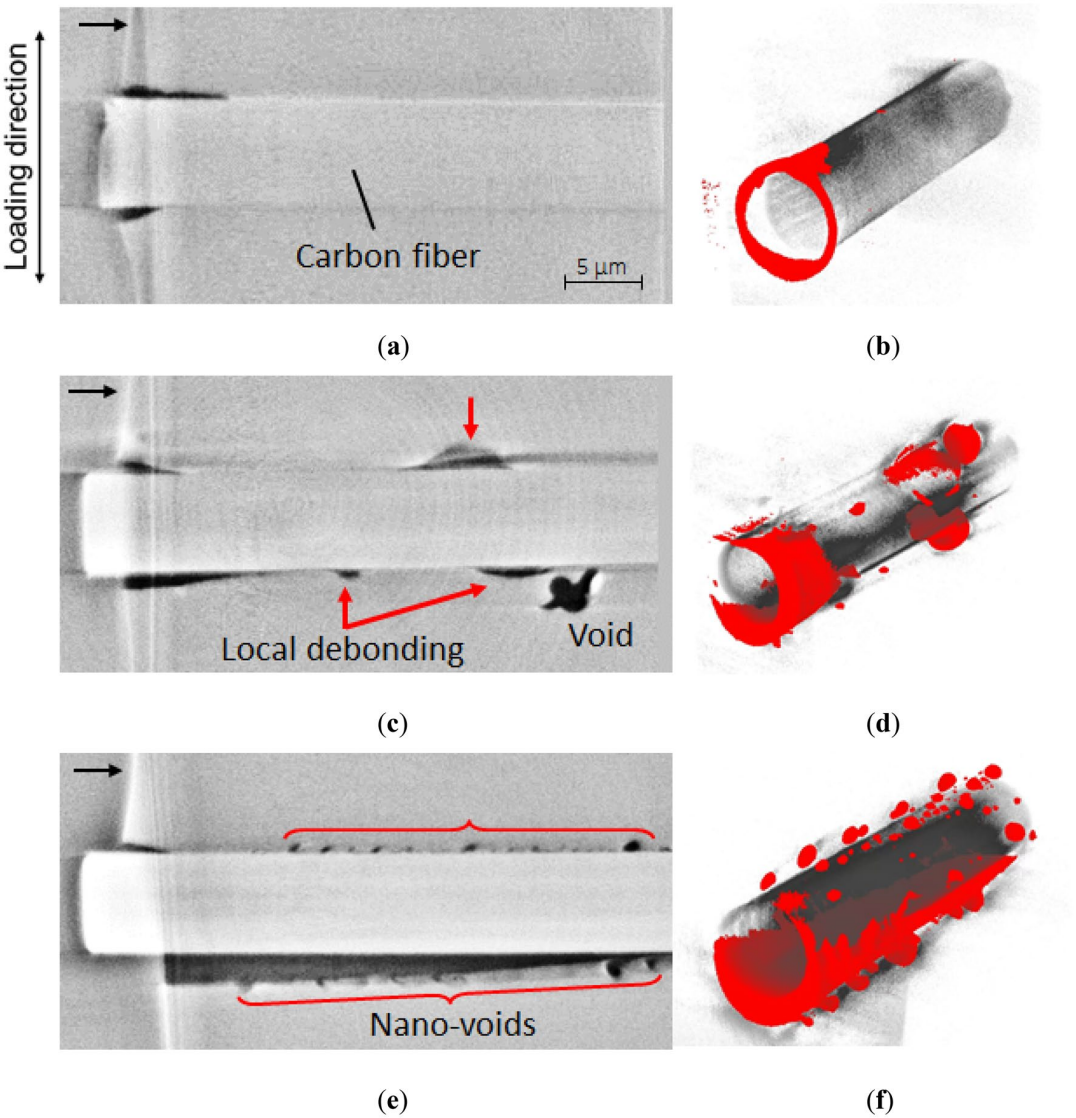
Reconstructed images recorded under stresses of (a, b) 30, (c, d) 40, and (e, f) 50 MPa by nano-CT (left) with the 3D image of the carbon fiber extracted using binarization process with empty spaces in the epoxy matrix represented in red. (right). The interfacial debonding was clearly captured from the sample surface even under 30 MPa. The local debonding isolated from the sample surface was also observed under 40 MPa. The interfacial debonding exceeded the field of view under 50 MPa, with an indication of the coalescence of voids prior to the separation from the carbon fiber.
